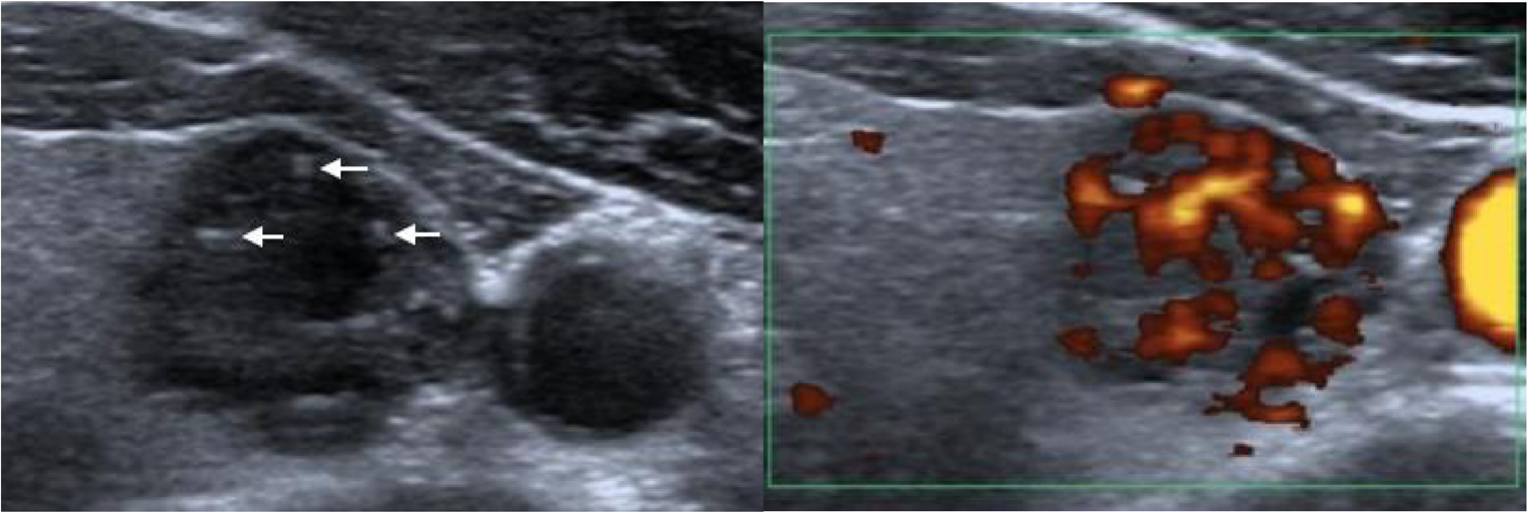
The thyroid nodule in the left lobe consists of colloids with their characteristic ‘comet-tail’ shadowing (arrows). However, it is hypo-echoic with an echo signal close to the adjacent strap muscle. Power Doppler shows marked intra-nodular vascularity. Multiple sinister characteristics of the nodule put it between U4 and U5, which require FNAC
